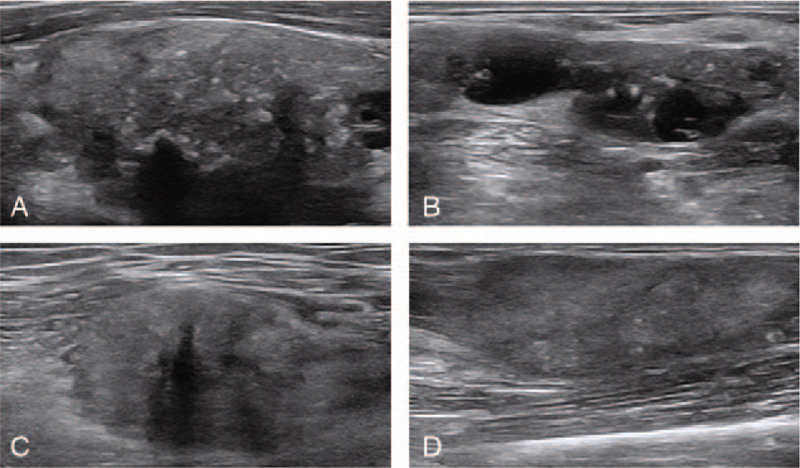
Ultrasound images. (A) A hypoechoic nodule with blurred borders can be seen on the upper right thyroid gland, and punctate calcification can be seen inside. (B) The right cervical lymph node has lost its hilum structure. There are solid hyperechoic and cystic areas in the lymph nodes. (C) A hypoechoic nodule can be seen in the middle part of the right thigh femoris, where punctate calcification is present. (D) A hypoechoic nodule can be seen in the middle part of the superficial flexor of the left forearm.
